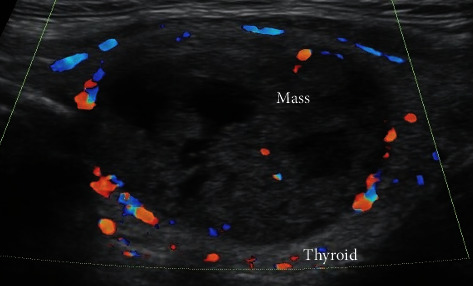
Ultrasonographic finding of the left thyroid nodule (28 mm × 19 mm in size).
